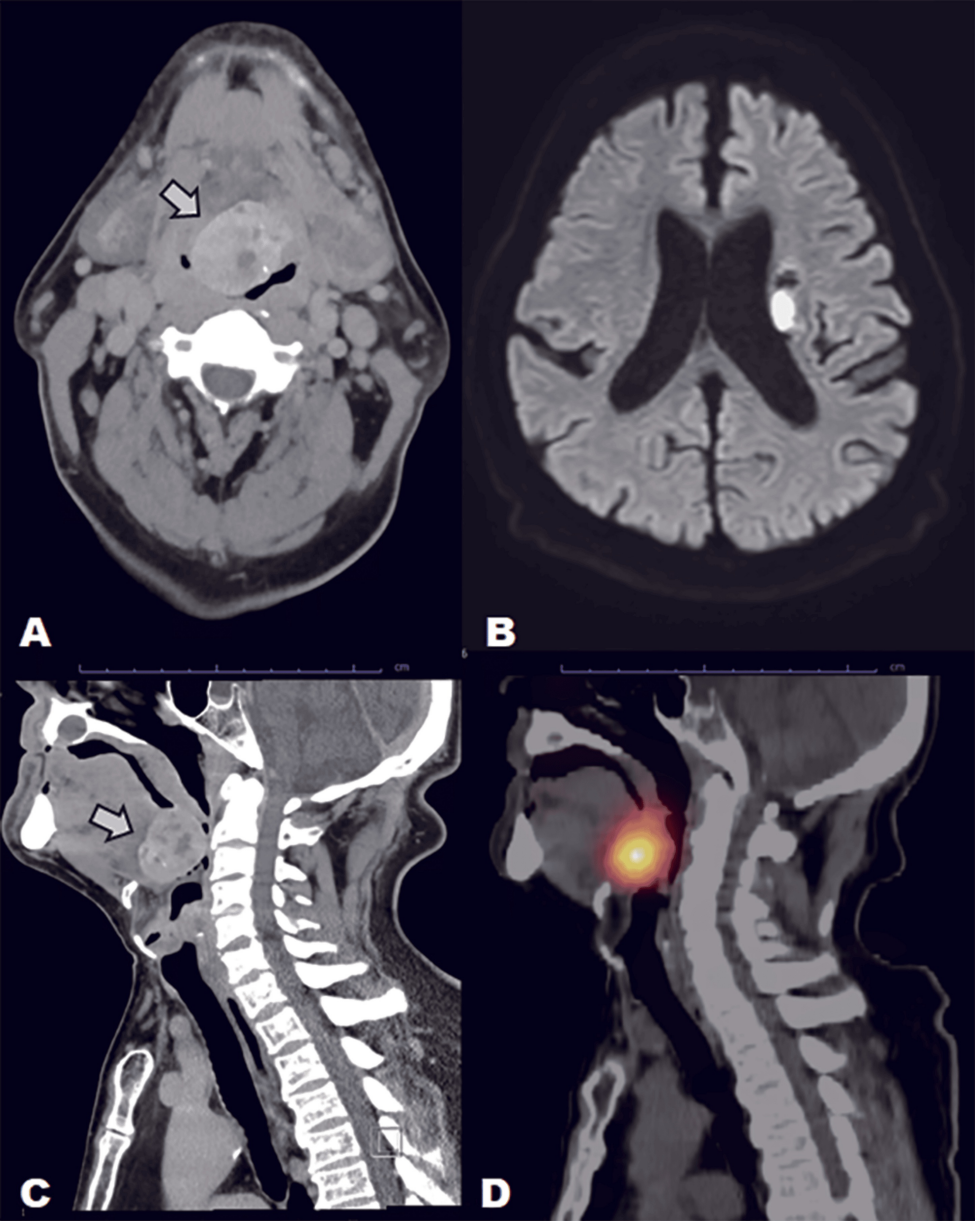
Preoperative multimodality imaging A. Axial noncontrast CT showing a 4.0 × 3.5 × 3.0 cm calcified tongue-base mass causing severe oropharyngeal airway narrowing; B. Axial DWI revealing acute infarction in the left corona radiata; C. Sagittal CT MPR demonstrating marked airway compression by the mass; D. I-123 scintigraphy showing intense uptake at the tongue base with absent cervical activity, confirming ectopic lingual thyroid
